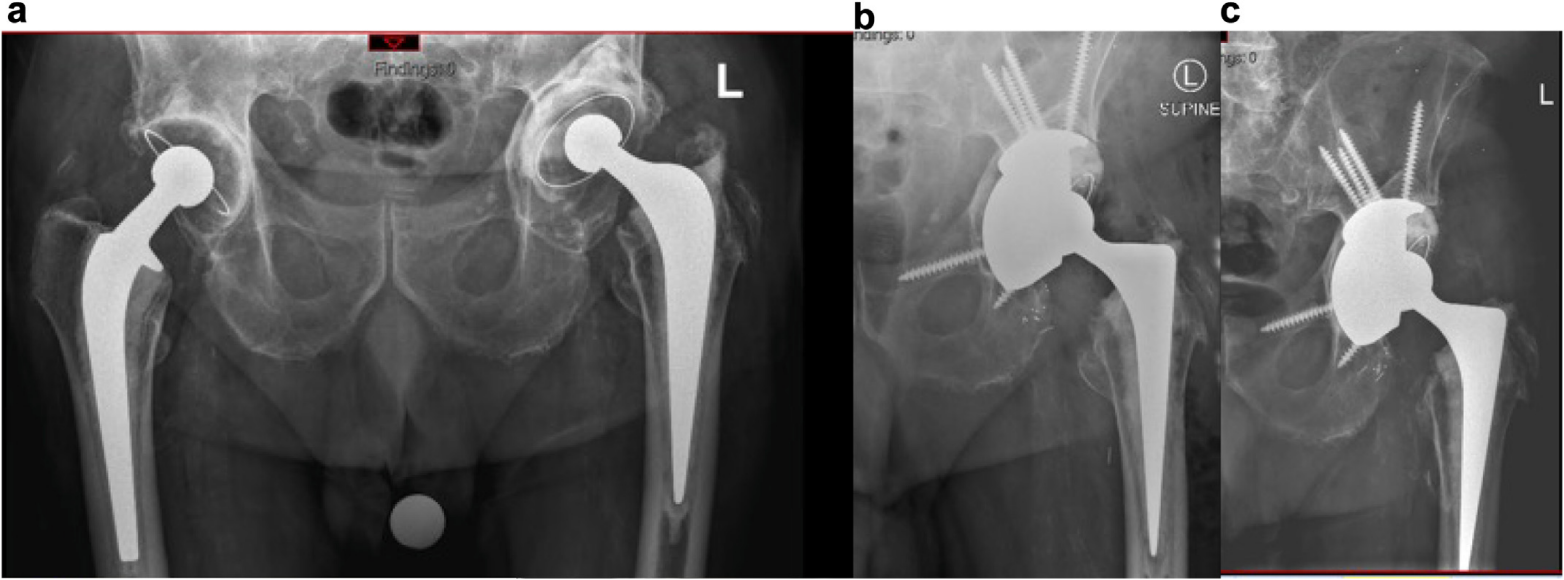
Exemplar radiograph images taken (a) preoperatively, (b) at 3 months postoperative, and (c) at 3 years postoperative from a patient undergoing a revision THA due to loosening and osteolysis. Note that the 3-year radiograph image is shown to demonstrate implant stability. THA, total hip arthroplasty.
